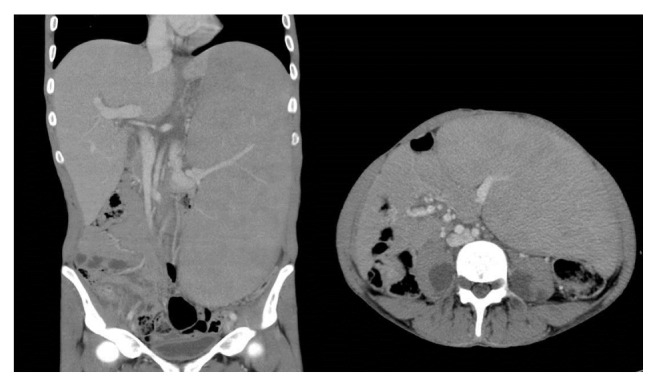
CT scan of the abdomen showing massive splenomegaly and bilateral plexiform neurofibromas along the psoas muscles.
